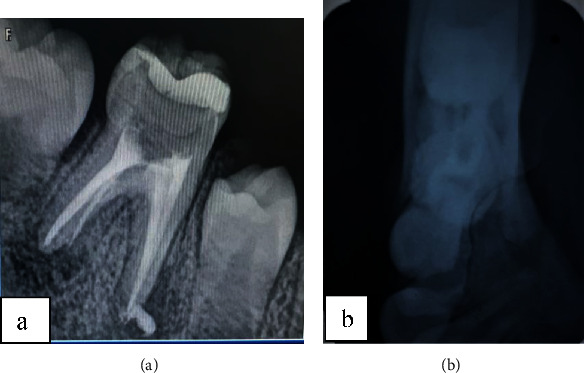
(a) Postoperative periapical radiograph after 4 months with signs of bone healing. (b) Occlusal radiograph after 4 months: partial disappearance of the periostitis.
